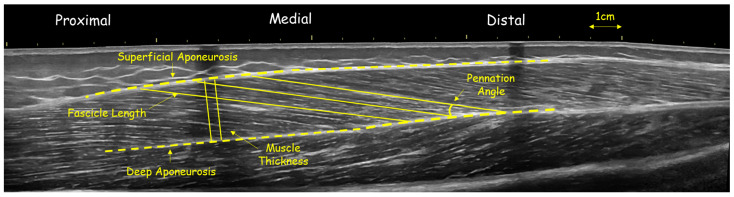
Panoramic ultrasound image of vastus lateralis of a volleyball athlete, showing fascicle length and angle and muscle thickness at mid-belly.
